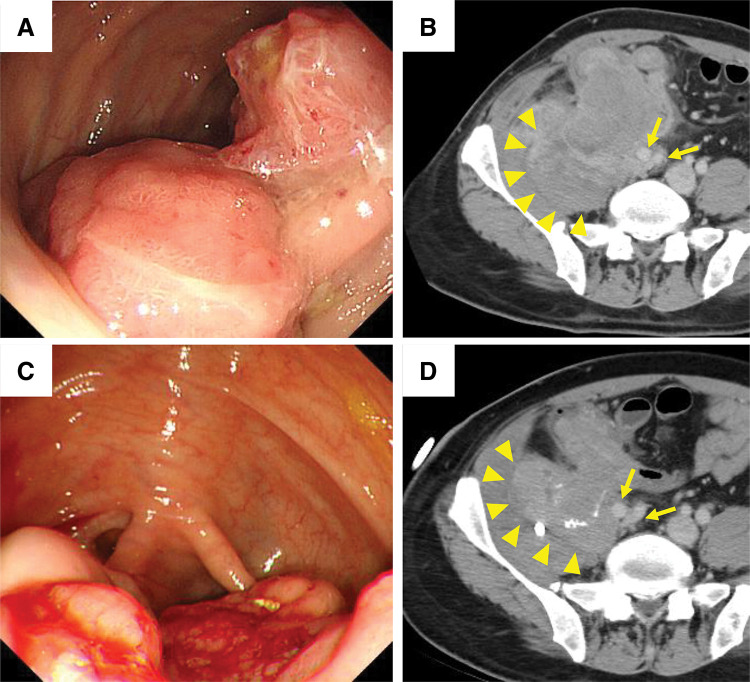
(A) Pre-chemotherapy colonoscopy shows a type 2 tumor in the cecum. (B) CT shows invasion of the external iliac vessels (arrows) and an abscess in the psoas muscle (arrowheads). (C) Post-chemotherapy colonoscopy shows shrinkage of the type 2 tumor. (D) CT shows shrinkage of the psoas muscle abscess (arrowheads); however, invasion of the external iliac vessels (arrows) remains.
